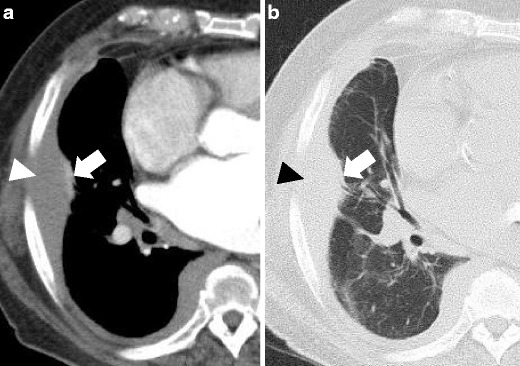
Primary effusion lymphoma; axial view contrast-enhanced CT scan: soft tissue (a) and lung (b) window images. A mild pleural effusion may be seen (*arrowhead*) with slightly hyperdensity, associated with a small lung consolidation with air bronchogram (*thick arrow*); no real pleural masses are recognised
